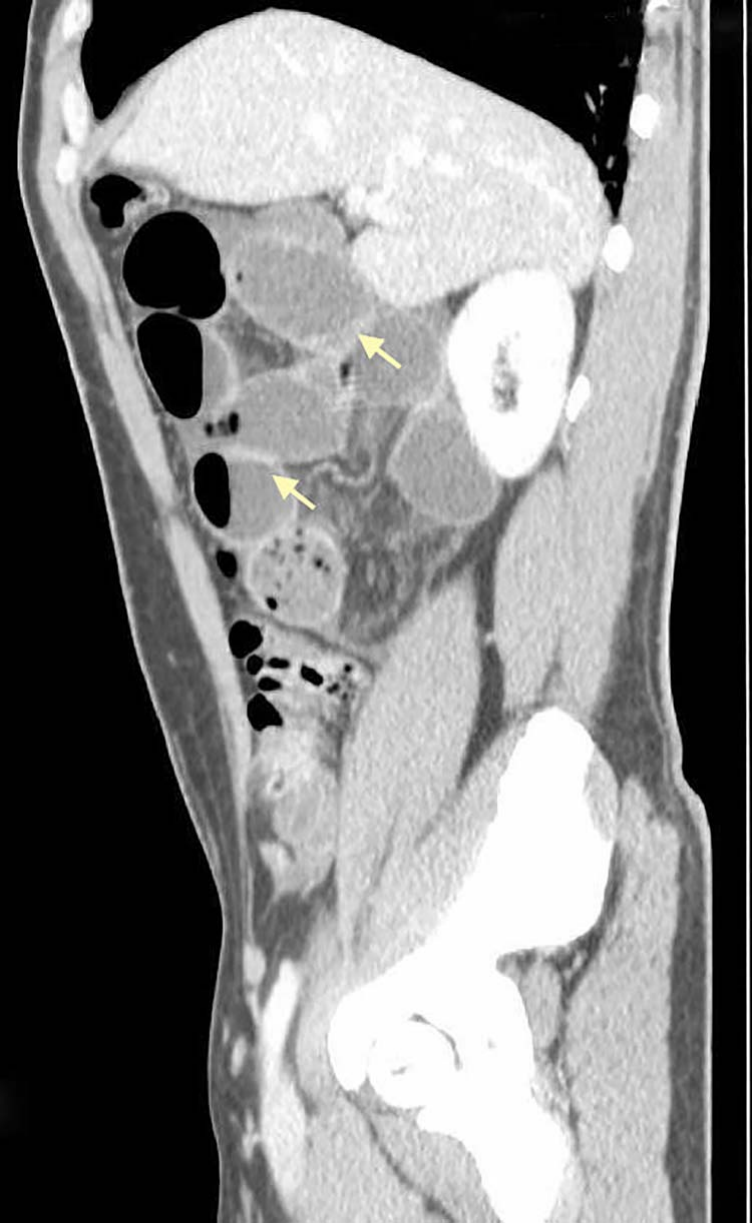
Sagittal CT scan shows small bowel distension with air/fluid levels (arrows).
